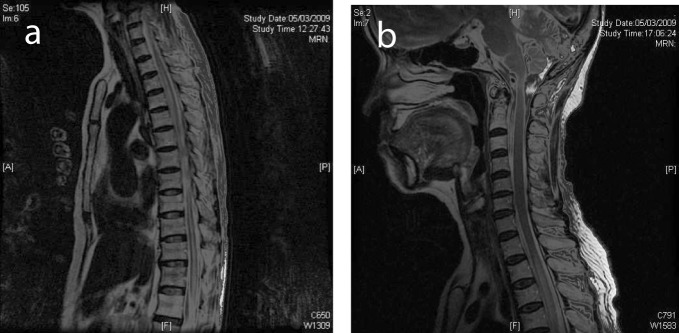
(a) Selected sagittal MR image of the thoracic spine on initial presentation showed normal cord signal. (b) Cervical cord on presentation showed normal MR signal.
